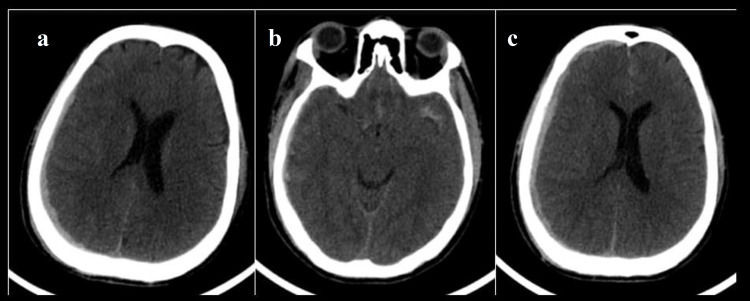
CT of a 47-year-old male patient following road traffic accident Initial admission CT shows (a) soft tissue subdural hemorrhage in the right parietal convexity causing mild effacement of the right lateral ventricle and leftward midline shift of 3 mm. Right holo-hemispheric edema was noted. Basal cisterns were normal - Rotterdam score 2. Sequential CTs after 24 hours show (b) left Sylvian cisternal subarachnoid hemorrhage and (c) increase in the thickness of subdural hemorrhage, however, midline shift did not increase. Right holo-hemispheric edema was noted. Basal cisterns were normal - Rotterdam score 3.
